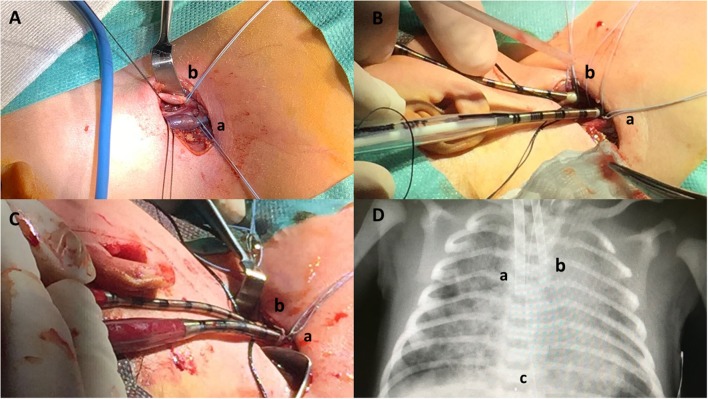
Neonatal veno-arterial Surgical cannulation site in the neck for respiratory ECMO: surgical steps. (A) Neck skin incision and isolation of internal jugular vein (a) and carotid artery (b). (B) Vascular cannulation. Drainage cannula (10 French) inserted in the jugular vein (a), inflow cannula (8 French) inserted in the carotid artery (b). (C) ECMO start. Drainage cannula (10 French) inserted in the jugular vein (a), inflow cannula (8 French) inserted in the carotid artery (b). (D) Chest X-ray. Drainage cannula (10 French) inserted in the jugular vein (a), inflow cannula (8 French) inserted in the carotid artery (b), and cannula tip (c).
